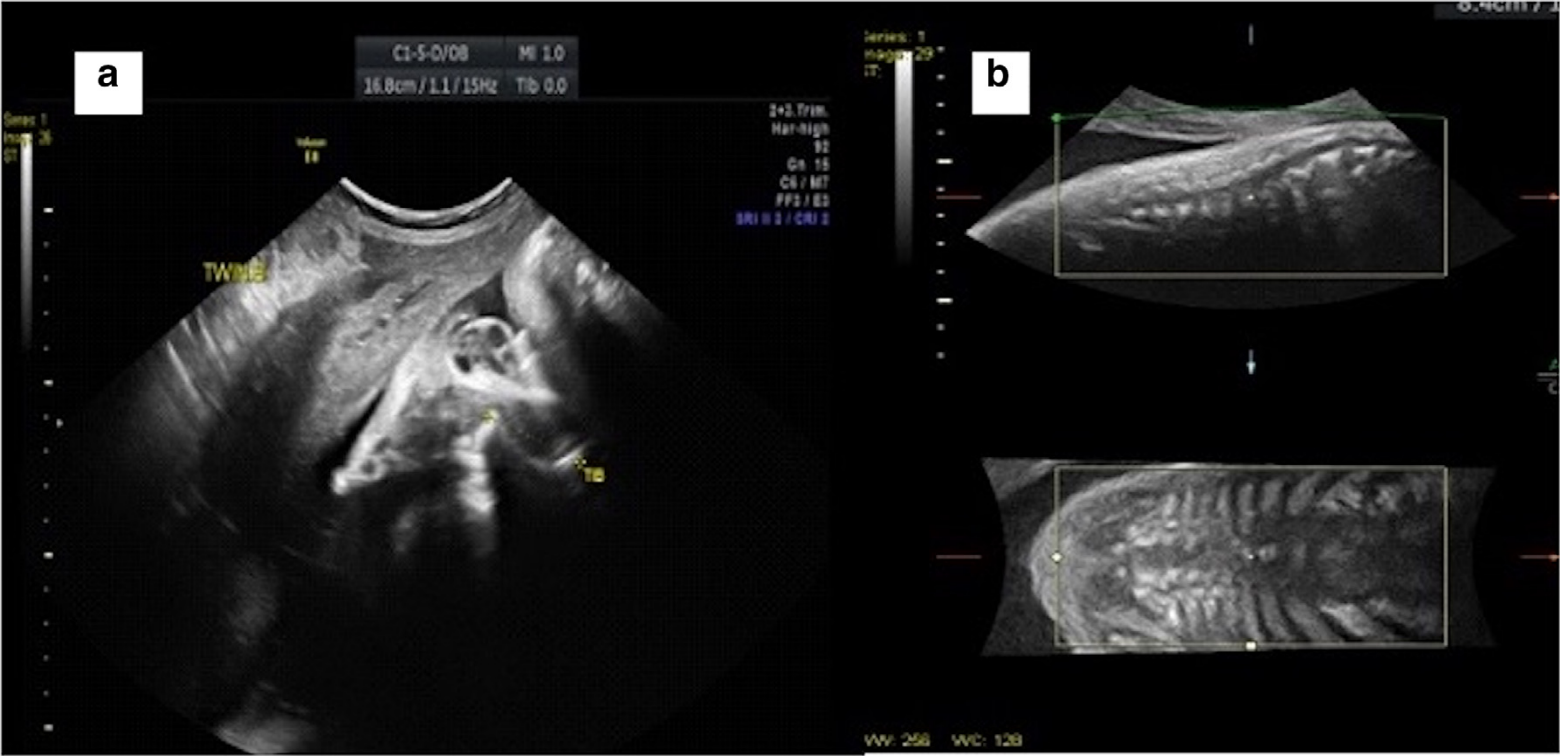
Fetal ultrasound (36 3/7 weeks): A. extreme bowing of tibia and B. vertebral segmentation defectsοMarked scoliosis with segmentation defects (Figure 1B), a small thorax and relative cardiomegalyοBone density was reported as normal, there were no detectable fractures
